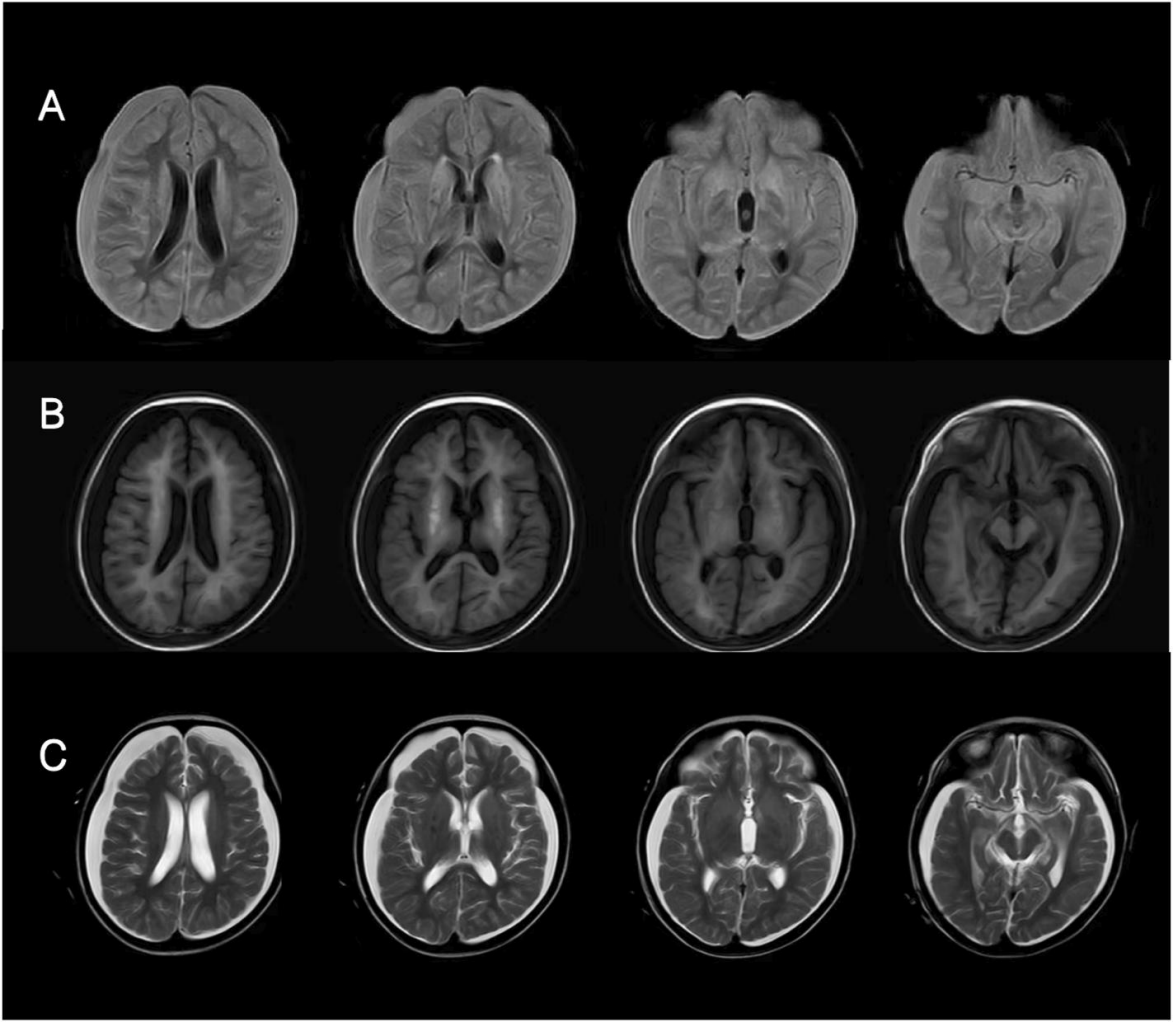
Brain MRI images of the patient in our hospital, (A) flair, (B) T1, and (C) T2.
